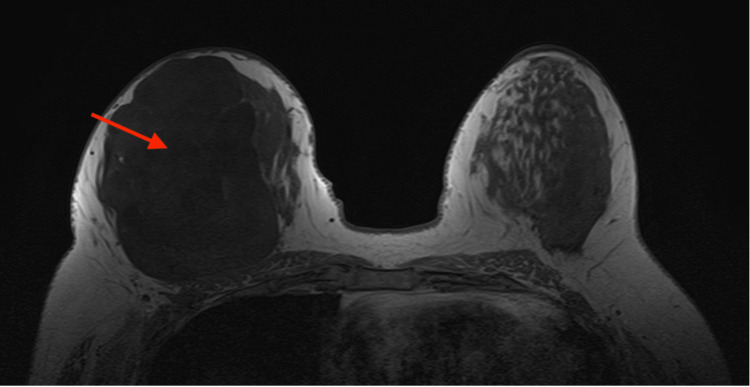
Preoperative breast MRI revealing a right-sided 10.9 x 9.4 x 11.7 cm heterogenous T2-enhancing lobulated mass that occupies the entire right breast.
